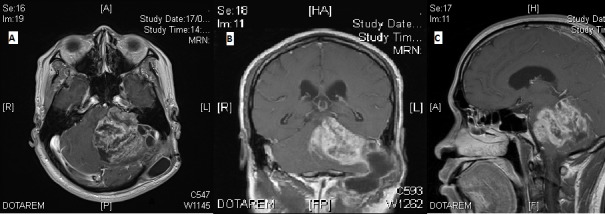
Magnetic resonance imaging at time of presentation A) Axial T1-weighted contrast-enhanced image; B) Coronal T1-weighted contrast-enhanced image; C) Sagittal T1-weighted contrast-enhanced image
